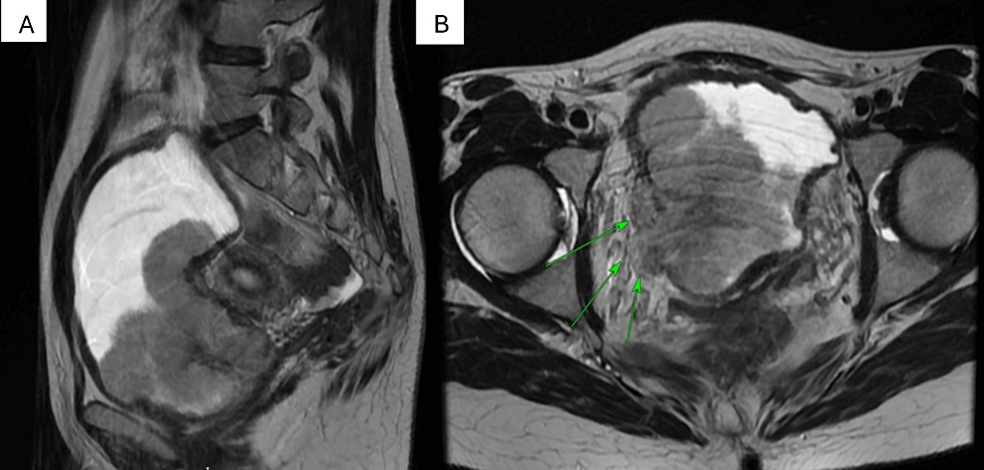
(A) Sagittal section of the MRI abdomen and pelvis. (B) Cross-sectional view of the bladder tumor with evidence of locally advanced bladder cancer extending into the anterior vaginal wall.
